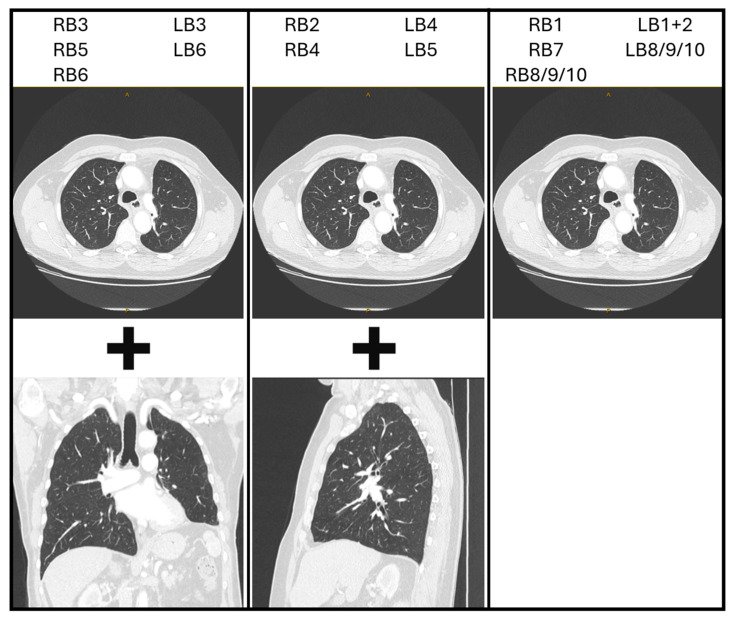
Potential CT image reconstructions for each segmental bronchus. RB = right segmental bronchi. LB = left segmental bronchi. For example, if navigating via the RB3 segmental airway, consider using axial images (with a horizontal flip ± 90-degree counterclockwise rotation) and coronal reconstructions.
